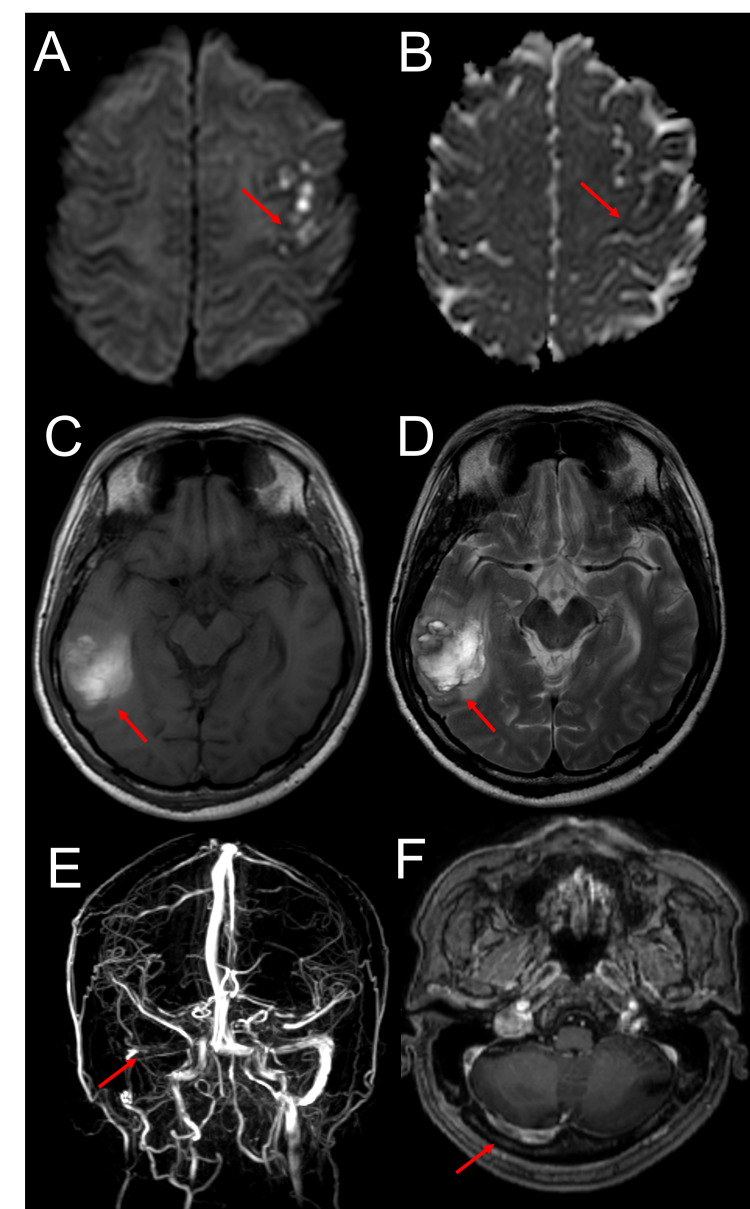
Restricted diffusion is seen at the left precentral gyrus with evidence of high signal on DWI sequence (A) and low signal on ADC (B) suggestive of venous infarction. A well-defined irregular lesion in the right temporal lobe, predominantly hyperintense on T1W (C) and T2W with thin surrounding hypointense rim (D). This is consistent with a combination of vasogenic oedema, cytotoxic oedema, and haemorrhage involving the right temporal lobe consistent with haemorrhagic venous infarction. On MRV, the right transverse and sigmoid sinuses are not seen, as shown by the red arrow (E). Post gadolinium T1 weighted image showing the intraluminal filling defect of the right transverse and sigmoid sinus consistent with dural venous sinus thrombosis (F).
